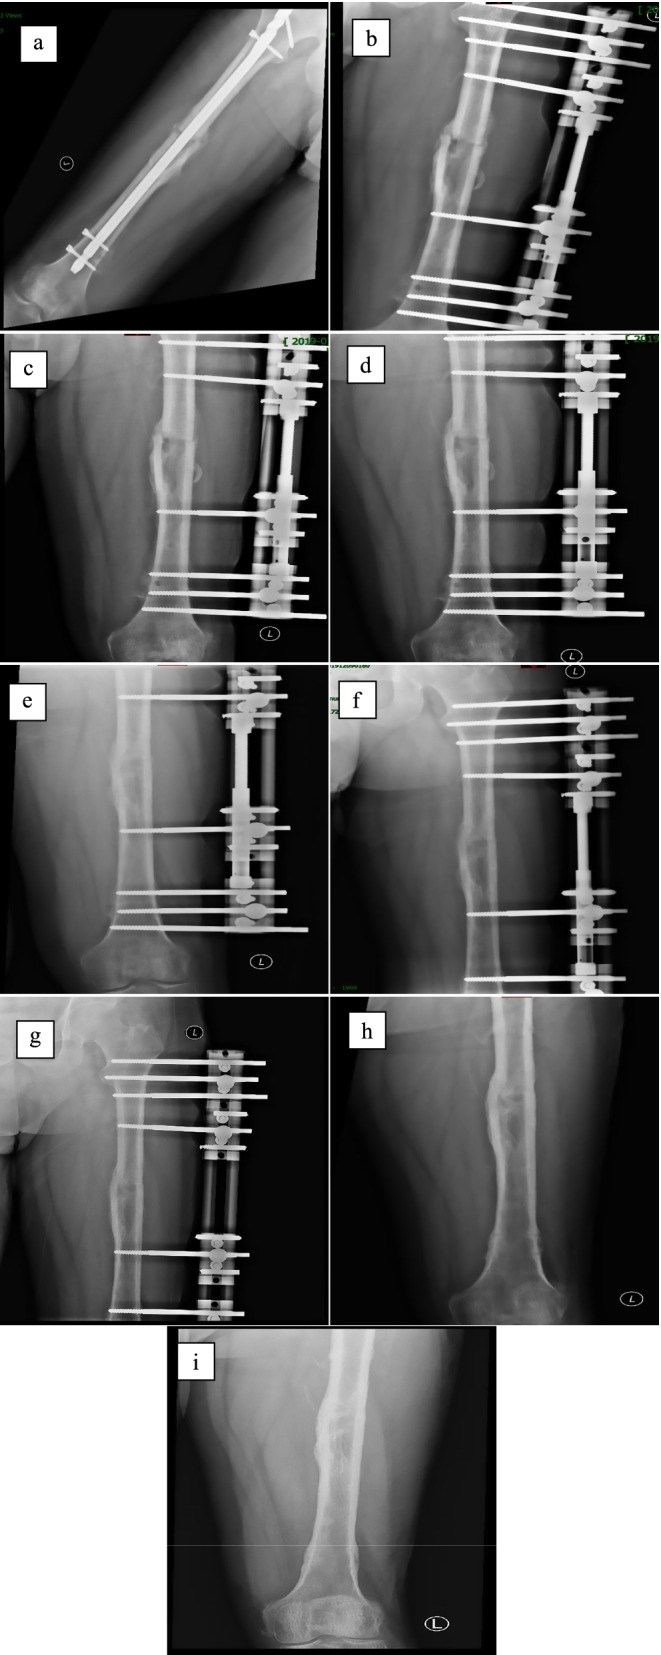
Typical case. A 37-year-old male patient with fracture of the femur due to traffic injury. Two months after intramedullary nail fixation, the patient developed swelling, ulceration, and purulent discharge on the medial femur. a preoperative radiographs showed intramedullary nail fixation of the femoral fracture. b one week after operation, radiographs showed that the intramedullary nail had been removed, the upper femoral medullary cavity was filled with artificial bone particles, and the external fixation was performed. c forty-five days after operation, bone callus can be seen at the fracture end, artificial bone particles were absorbed. d four months after operation, the callus grew well and the artificial bone particles were absorbed completely. e six months after operatian, the callus grew well and the external fixation was performed. f seven months after operation, the callus grew well and the external fixation was performed. g eight months after operation, the callus grew well and the extermal fixation was performed. h eleven months after operation, the fracture was healed, and the external fixator was removed. i sixteen months after operation, radiographs showed that the fracture healed well
